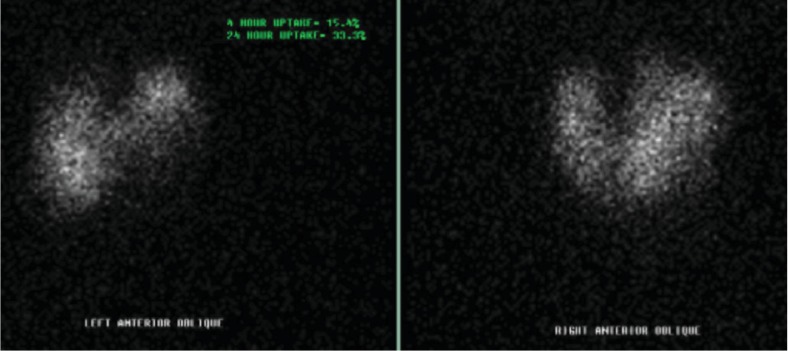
Thyroid uptake (I-123) scintigraphy showing elevated radioactive iodine uptake at 4 and 24 hours consistent with hyperthyroidism and a cold defect in the inferior pole of an enlarged left lobe for which thyroid carcinoma cannot be excluded.
